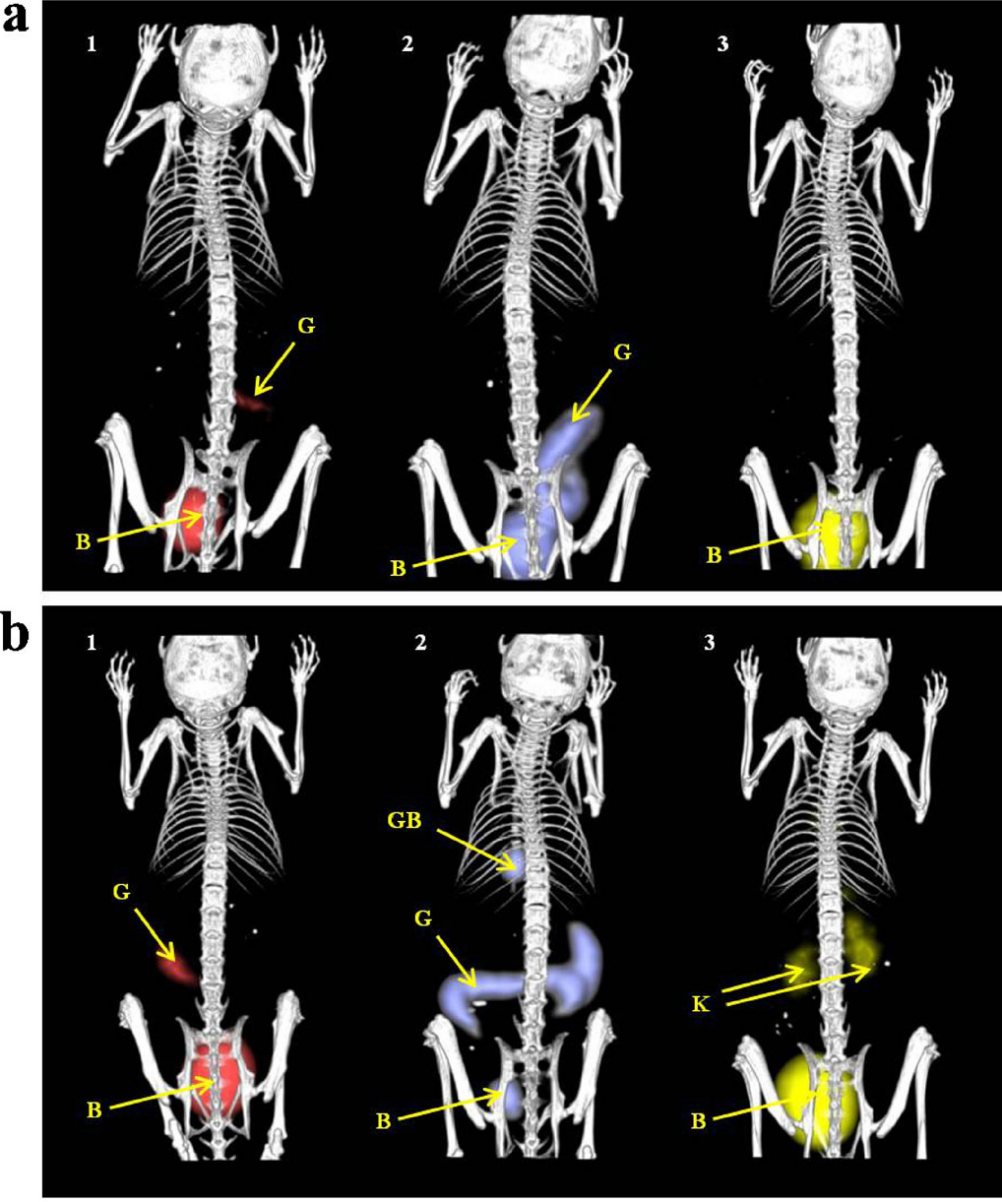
3D volume rendered images of static μPET/CT imaging of [^68^Ga]siderophores (a—TAFC = 1, FOXE = 2, FOXB = 3) and [^89^Zr]siderophores (b—TAFC = 1, FOXE = 2, FOXB = 3) 90 min p.i. (supine position; injected dose, 5–10 MBq; anaesthesia, 2 % isoflurane; scan duration, 5 min PET scan followed by 20 min CT scan; *B*—bladder, *G*—gastrointestinal tract, *GB*—gall bladder, *K*—kidney).
